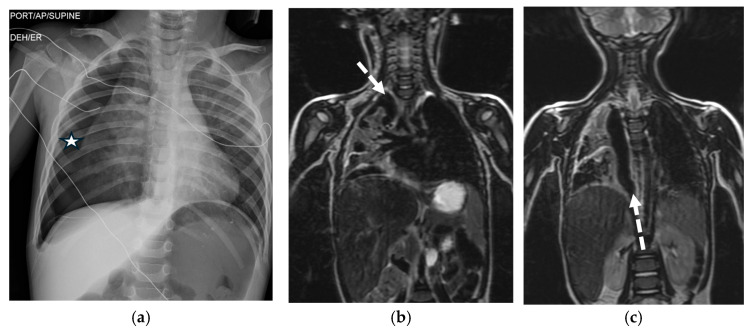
3-year-old boy presents after a motor vehicle accident. Frontal chest radiograph (a) demonstrates moderate-to-large right pneumothorax (white star) with associated partial atelectasis of the right lung. Coronal heavily T2W MR images obtained during thoracic spinal MRI examination (b,c) again show very-low-signal-intensity right pneumothorax (dashed white arrow) along with increased signal intensity of partially atelectatic right lung. Although MRI is not a common way to diagnose a pneumothorax, it can be used to identify one when encountered incidentally.
